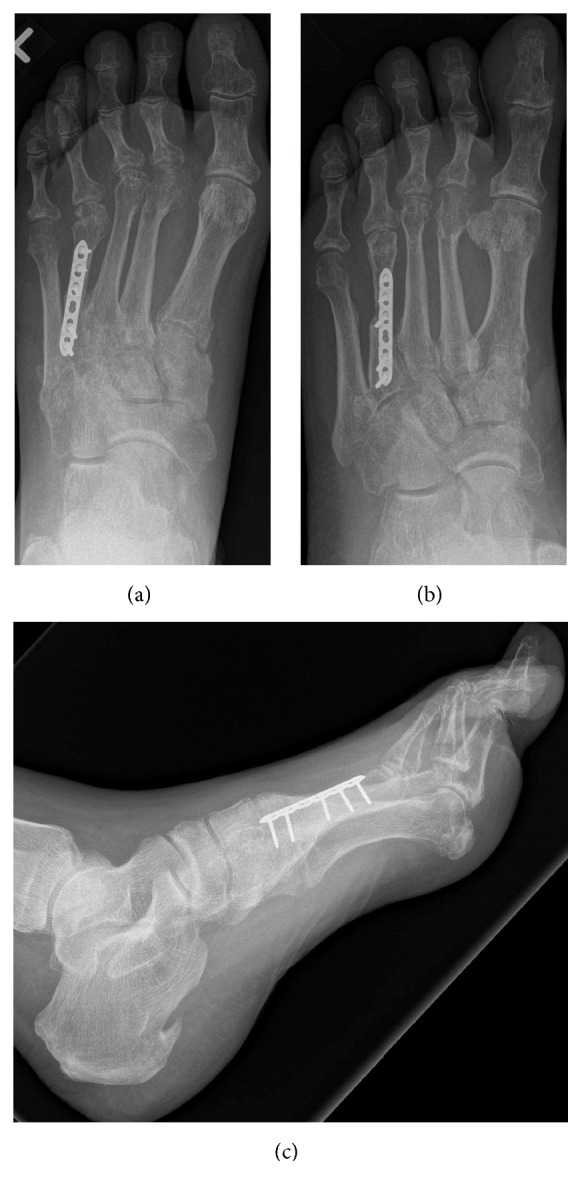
Final dorsoplantar (a), oblique (b), and lateral (c) radiographs showing fusion at fracture site, shortening of fourth MT, and maintained reduction at the fourth MTP joint.
